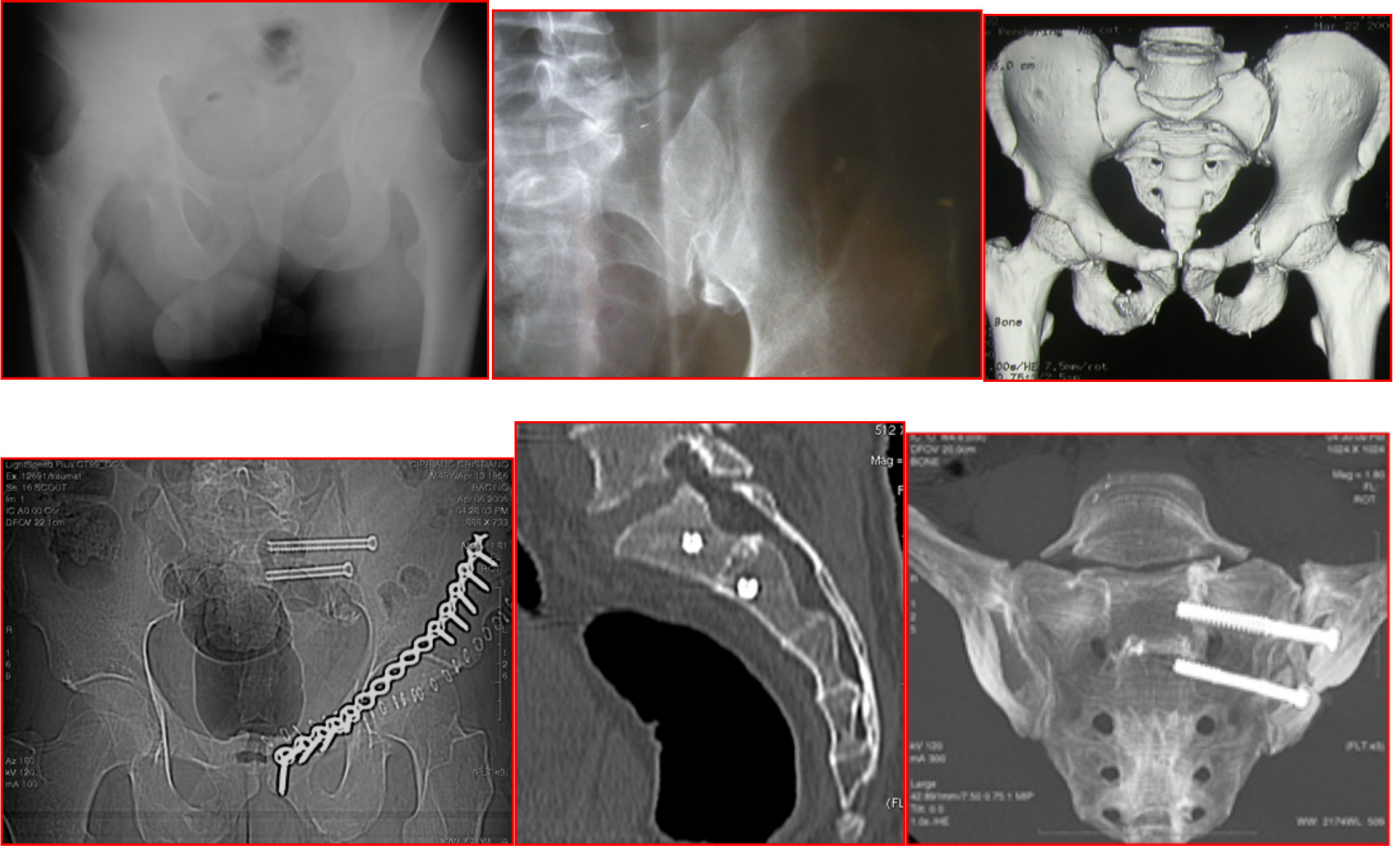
Case of a male patient (49 years old) with C1 Tile fracture, left ileopubic rami fracture and left S-I joint instability following a motorbike accident (top three pictures: AP X-ray view and pelvis 3D CT reconstruction). The patient was initially treated with S-I joint reduction and fracture reduction with plate and screws by anterior approach (bottom-left picture). This was followed (4 days following the initial surgical procedure) by surgical stabilization of the S-I joint with two percutaneous CT-guided cannulated screws within S1 and S2 vertebral body (sagittal and coronal CT-scan views).
